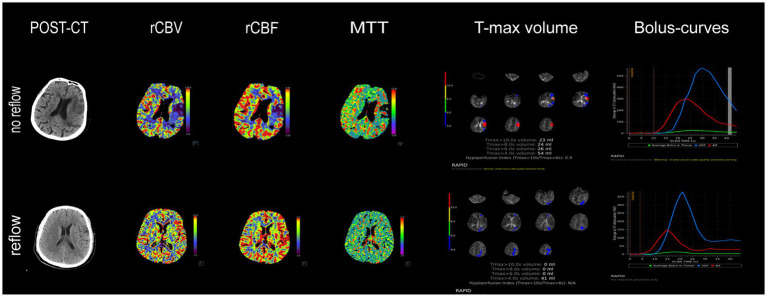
Representative CT perfusion cases of with and without reflow. No-reflow identified on CT perfusion and normal follow-up perfusion imaging for comparison.
